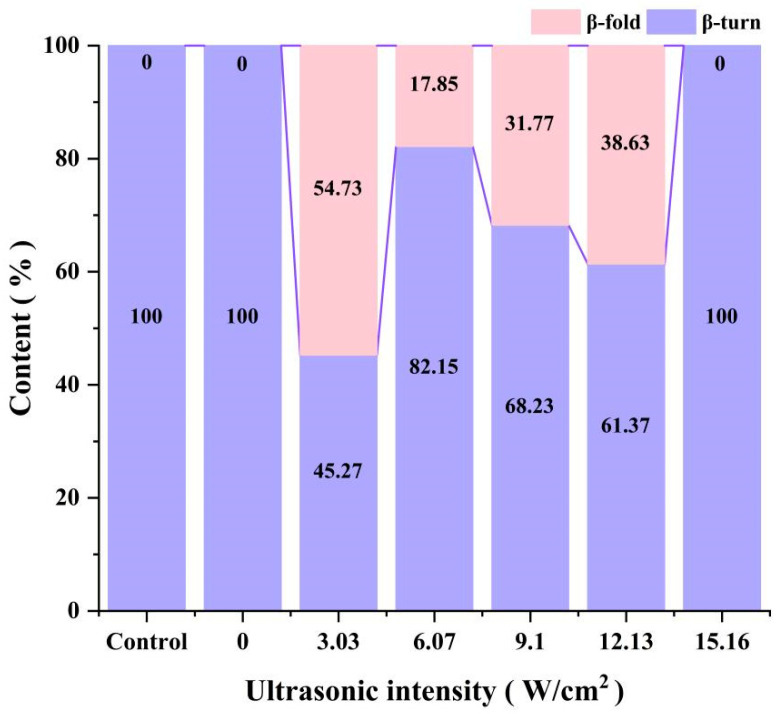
The effect of ultrasonic intensity on the secondary structure of CPP during UA acid extraction.
